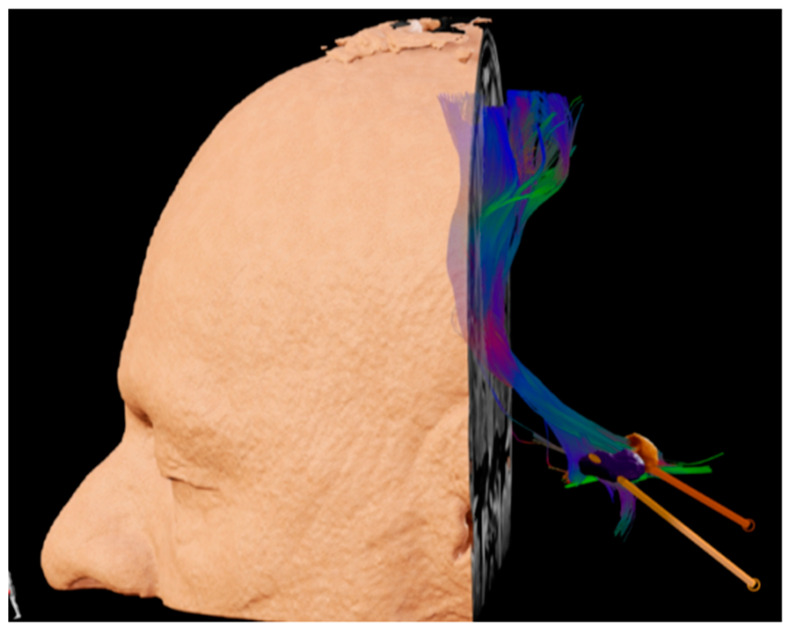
Post-operative image control of dentate nucleus DBS. Sagittal T1-weighted MRI reconstruction of DBS electrodes in the left (purple volume) and right (orange volume) dentate nucleus, with a close relation to the ascending dentato-rubro-thalamic tract. Reconstructions and tractography were performed with Elements Software (Brainlab AG, Munich, Germany).
